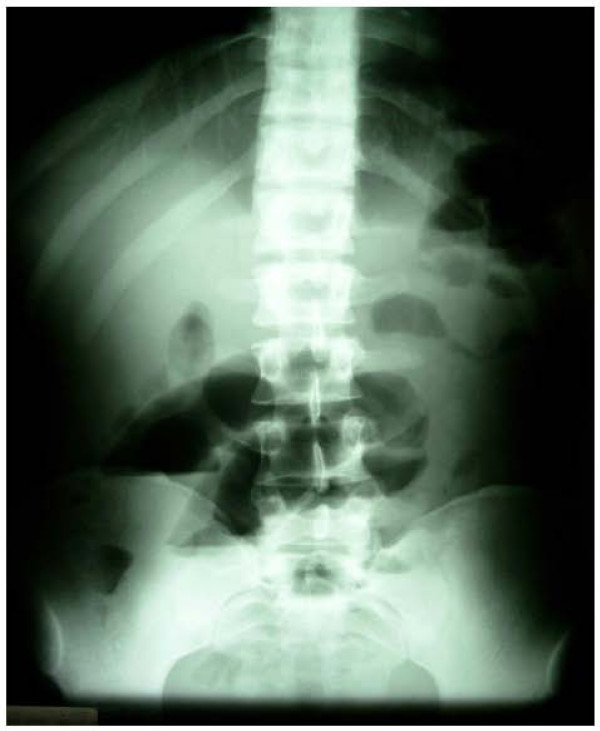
X-ray shows multiple loops of dilated small bowel.with air- fluid levels.
